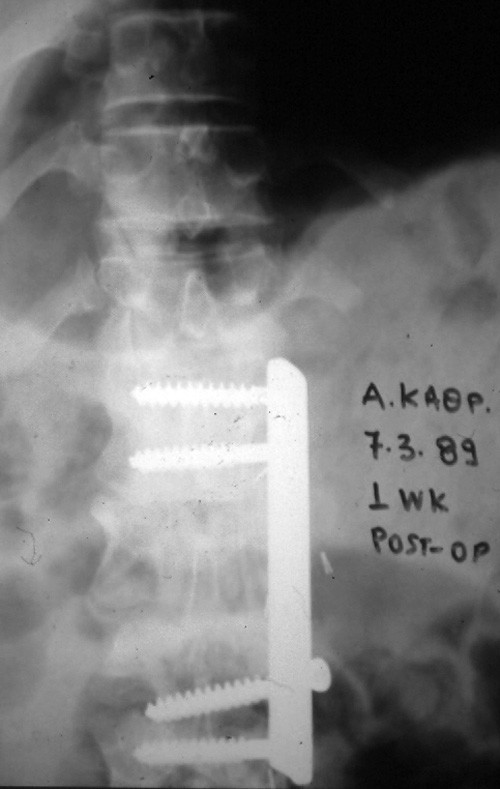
Postoperative anterior-posterior radiograph showing stabilization of the spine with a plate and screws.
